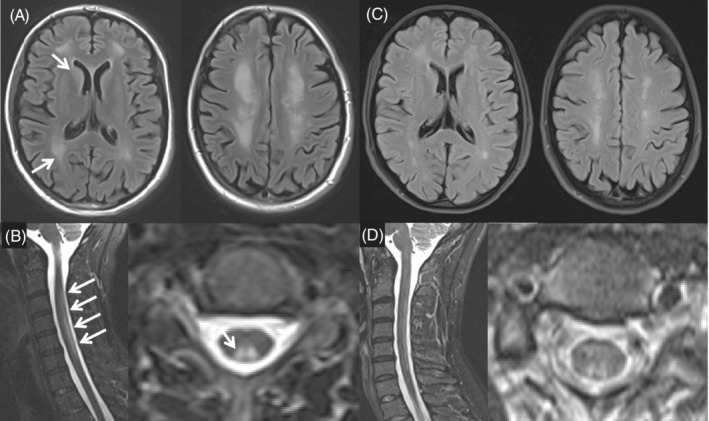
T2/FLAIR MRI Images of the brain and spinal cord of the proband patient before and after treatment. Panel A, T2/FLAIR images of the brain before treatment: note the fairly confluent hyperintensity involving periventricular white matter of the supratentorial brain. Putamen and head of caudate (arrows) are diffusely hyper‐intensive. Similar changes are shown on the medial occipitotemporal region. Panel B, T2/FLAIR images of the spinal cord before treatment: note the increase in hyperintensity in the dorsal columns from C2 to middle C6 levels and from T7 to T10 levels (arrows). No apparent loss of spinal cord volume was noted. Panel C, T2/FLAIR images of brain after treatment. Note the increased T2/FLAIR hyperintensity signals involving periventricular white matter. These lesions appear less prominent as compared to that in Panel A. The degree of increased T2/FLAIR signals involving the caudate and putamen are much less conspicuous as compared to that in panel A. Panel D, T2/FLAIR images of spinal cord after treatment. Note the increased T2 signal involving the posterior columns of the spinal cord spanning C2‐C7 levels. The foci of T2‐weighted hyperintensity signals appear much better circumscribed and smaller in cross section appearance as compared to that in panel B
